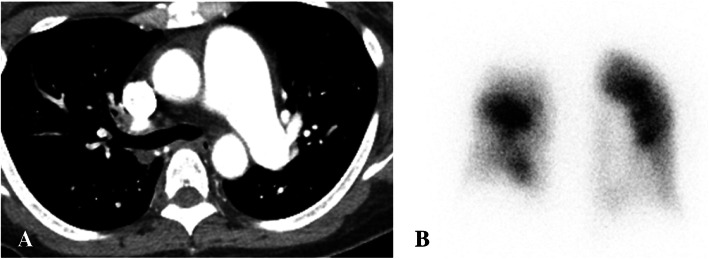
Pre-balloon pulmonary angioplasty images. a Contrast-enhanced computed tomography showed no emboli in the pulmonary arteries. b Lung perfusion scan showed multiple blood flow defects
